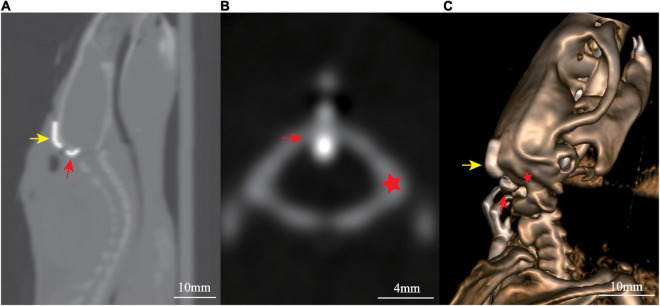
Post-surgical computed tomography (CT) scan showed the localization of cannulation: (A) sagittal view, (B) axial view, and (C) three-dimensional reconstruction. Yellow arrow marks the fixing segment, while red arrow marks the puncture segment. The red star marks the occipital bone.
